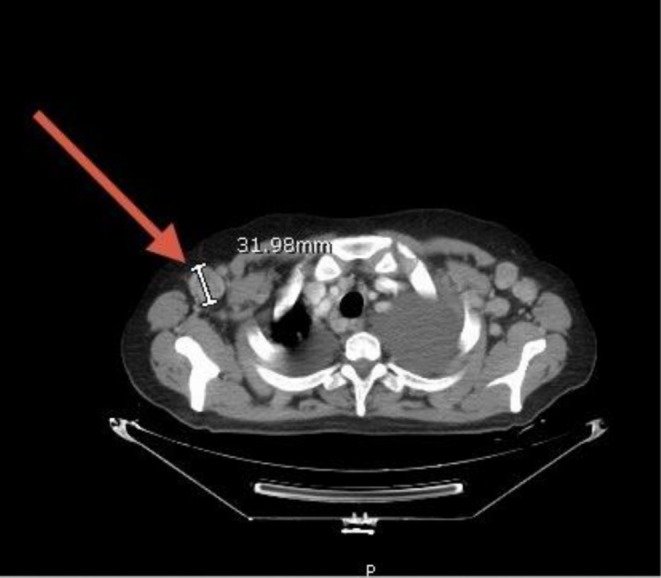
Axillary lymphadenopathy. Contrast‐enhanced CT of the axillary regions showing bilateral enlarged lymph nodes, indicating generalized nodal involvement.
